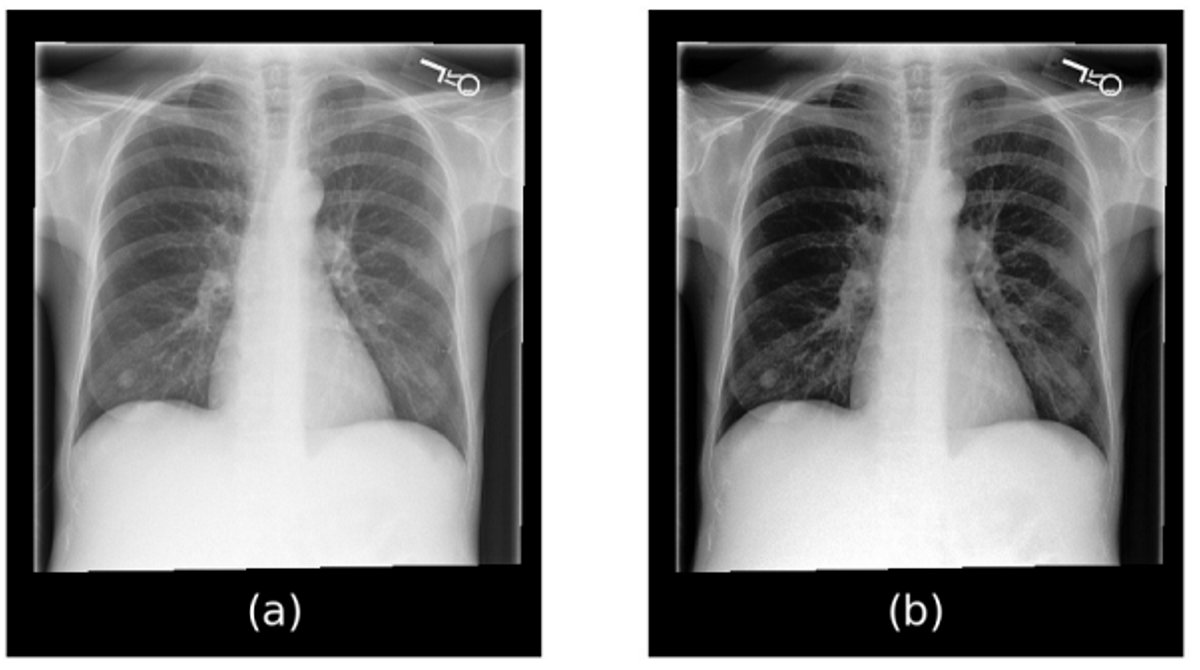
Chest x-rays in the Medical Information Mart for Intensive Care-Chest X-ray data set.
